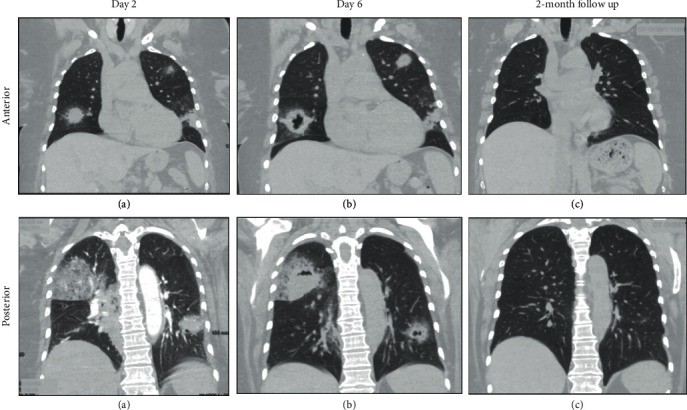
CT chest progression of pulmonary disease in anterior and posterior cuts of the coronal plane demonstrating rapidly cavitating disease. Chronology: (a) 2 days after admission; (b) 6 days after admission; (c) 2-month follow-up imaging.
